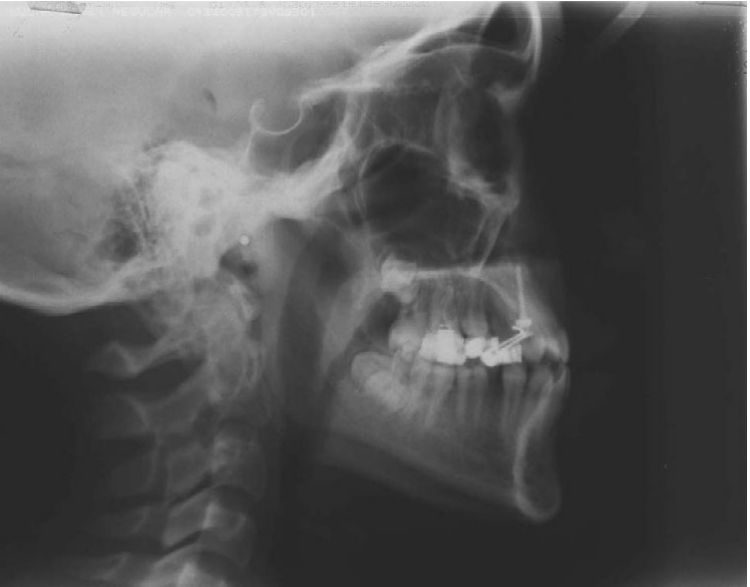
After distalization cephalometric radiograph of the patient.
